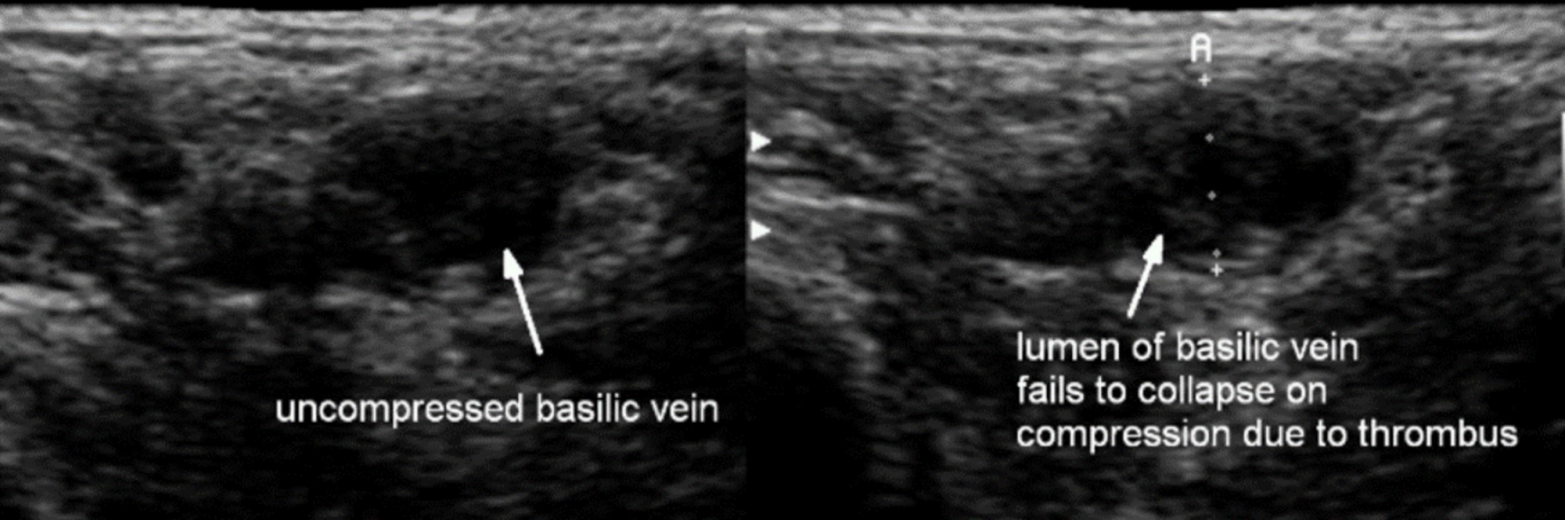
Venous Duplex Scan of the Upper Extremity The panel on the left shows a basilic vein that is not compressed by the ultrasound probe. In the right panel, the vein is compressed by the ultrasound probe; however, due to the presence of a clot within the lumen of the vein, the lumen is not collapsible (arrow).
